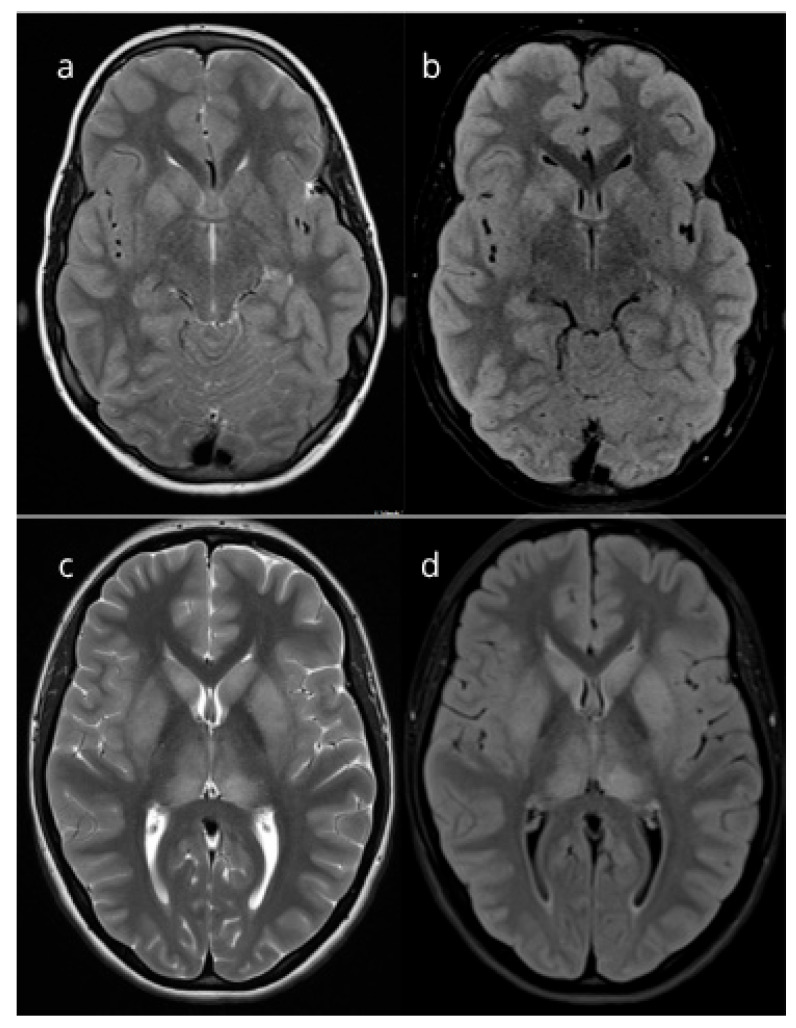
Magnetic resonance imaging (MRI) findings in patients. Axial T2-weighted (a) and axial FLAIR (b) MRI images of Patient 1 show bilateral asymmetrical hyperintensities in the head of the caudate nuclei and the right putamen, suggestive of encephalitis. Axial T2-weighted (c) and axial FLAIR (d) MRI images of Patient 2 demonstrate bilateral symmetrical hyperintensities in the basal ganglia and thalami, also suggestive of encephalitis.
